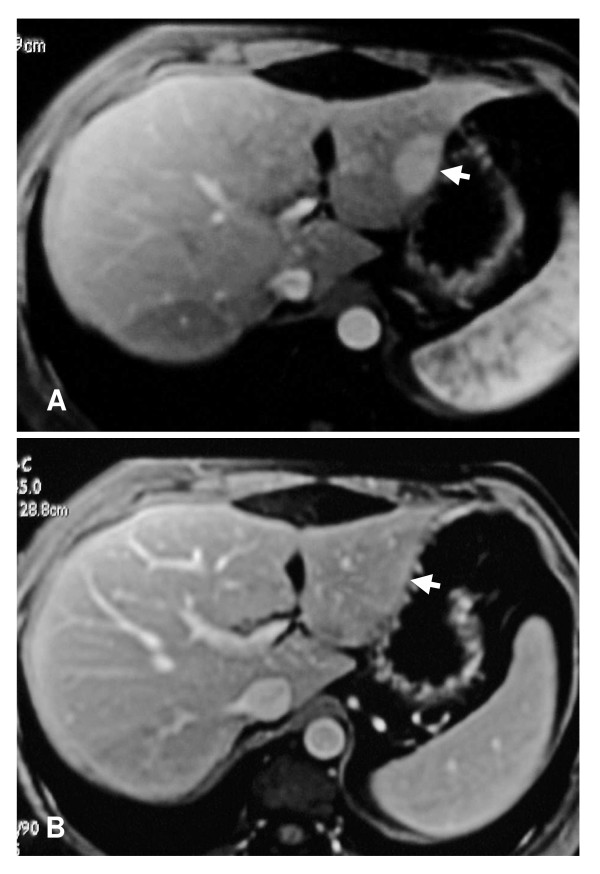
Contrast-enhanced T1-weighted images. They show strong enhancement of the lesion (white arrow) at arterial phase (A) and isointense signals relative to liver parenchyma at portal venous phase (B).
